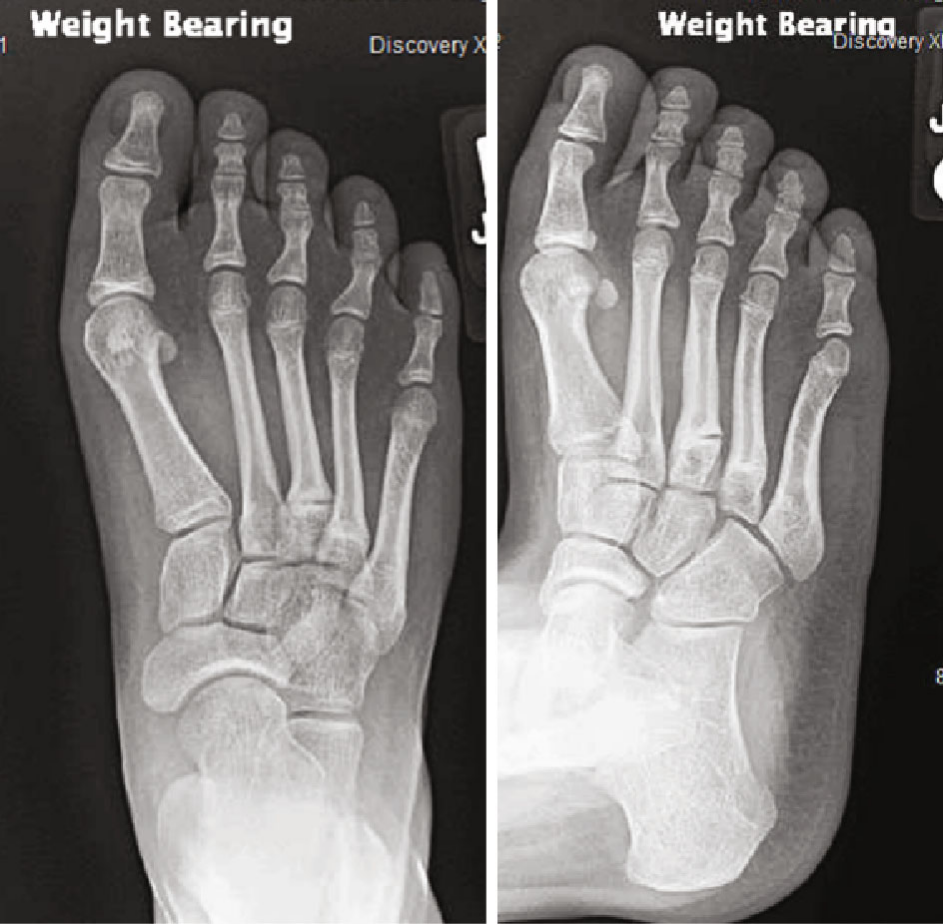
Radiographs of the right foot showing chronic incomplete fractures of the shafts of the third and fourth metatarsals.
